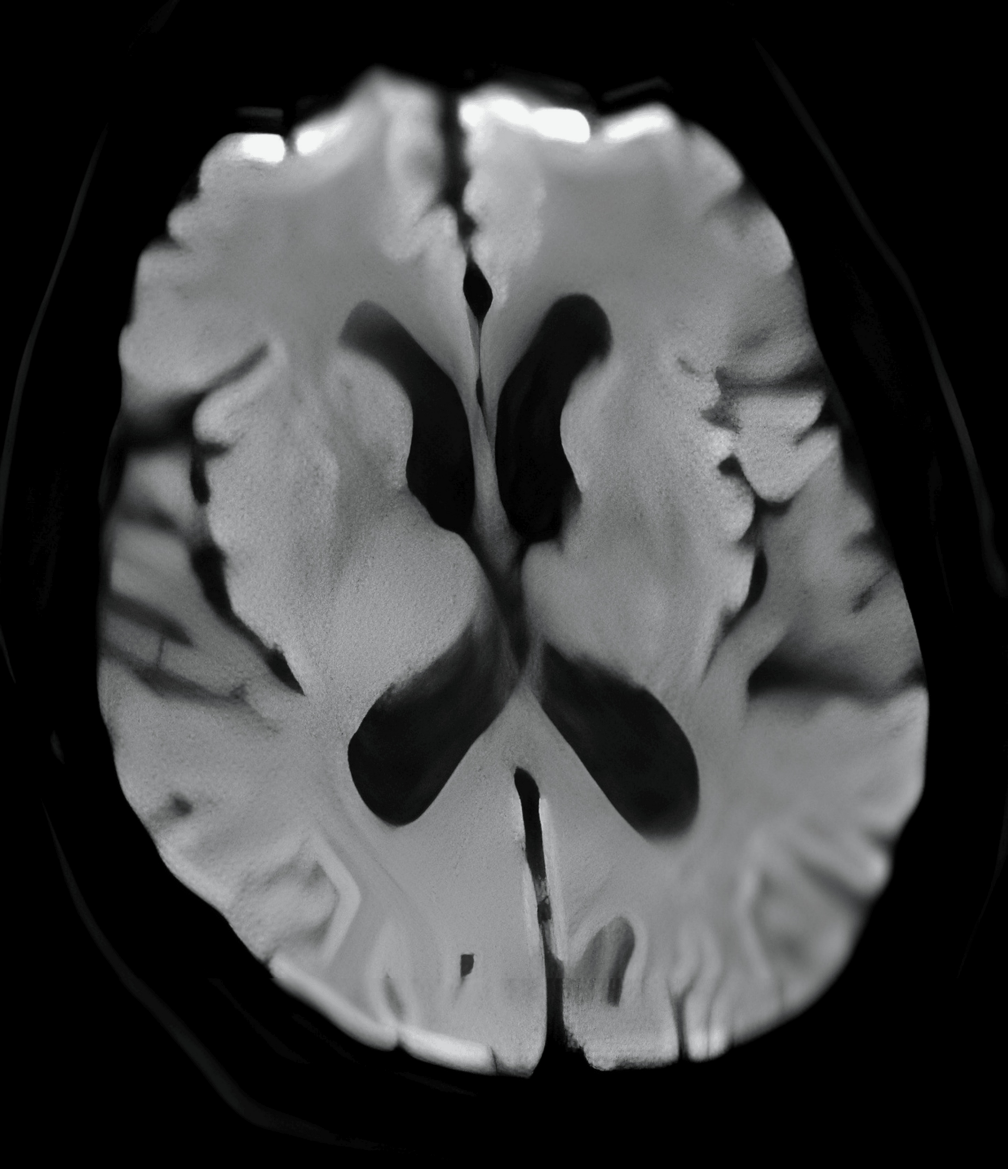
MRI of the head using a diffusion-weighted imaging sequence, demonstrating no evidence of restricted diffusion or acute infarction.
